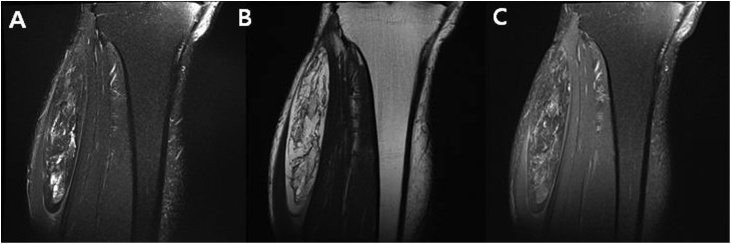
MRI showing a well defined ossified mass in the peroneous muscle. Coronal T2-weighted Fat-suppression (A), Coronal T2-weighted (B), Coronal T1-weighted Fat-suppression contrast enhanced (C).
